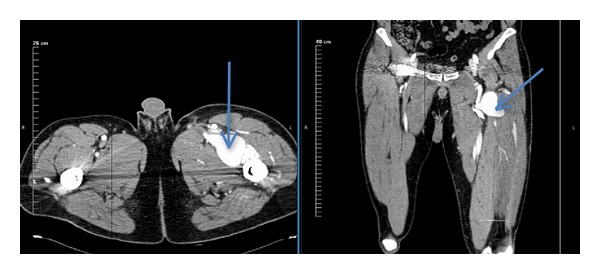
CT angiogram showing the left profunda femoris artery pseudoaneurysm.
